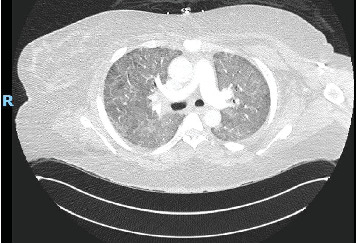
Computed tomography of the chest with angiography. Worsening diffuse ground glass opacities are present concerning for edema, inflammation, or hemorrhage. There is a similar degree of bronchiectasis compared to prior.
